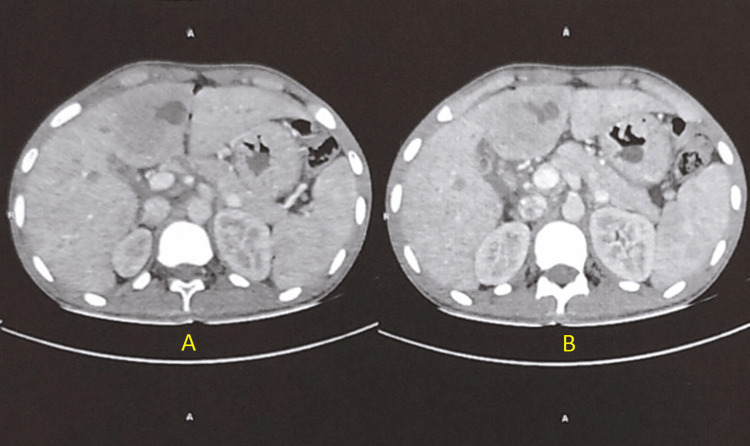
Sagittal section of CECT abdomen and pelvis showing a dilated stomach with fluid residue and multiple liver lesions Panel A shows well-defined oval cystic lesions in segment IVB and segment III of the liver. Panel B shows a dilated stomach with fluid residue. CECT: Contrast-enhanced computed tomography
